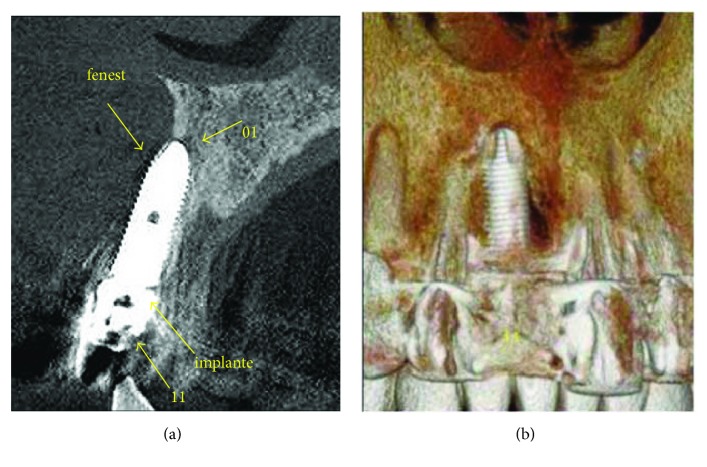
Radiographic analysis ten days after the trauma. (a) The tomographic image registers the implant in an inadequate three-dimensional position. (b) Loss of vestibular wall that had been rebuilt.
